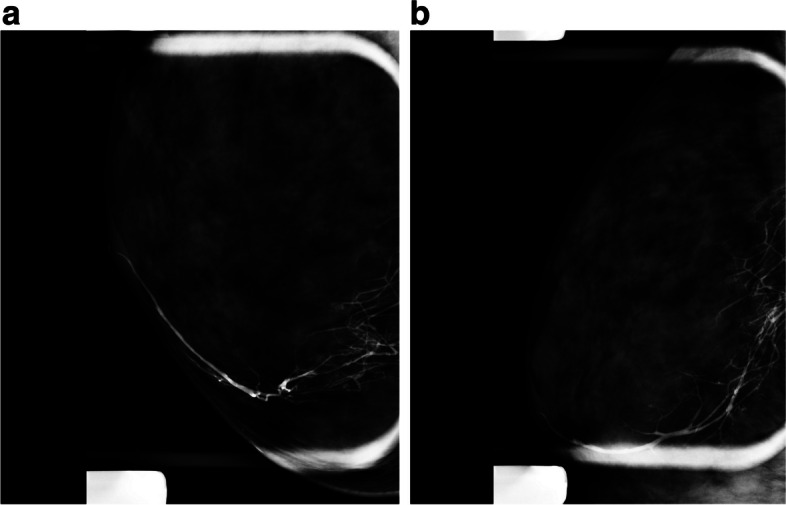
A 48-year-old woman presented with right breast brown color nipple discharge. Ductography radiographs show adequate opacification of the ducts. There is no evidence of a filling defect to suggest the cause of nipple discharge. There are areas of mild ductal dilatation, some anterior pruning, and mild dilation of the terminal ductal lobular unit suggestive of fibrocystic change. The nipple discharge stopped within 6 months
